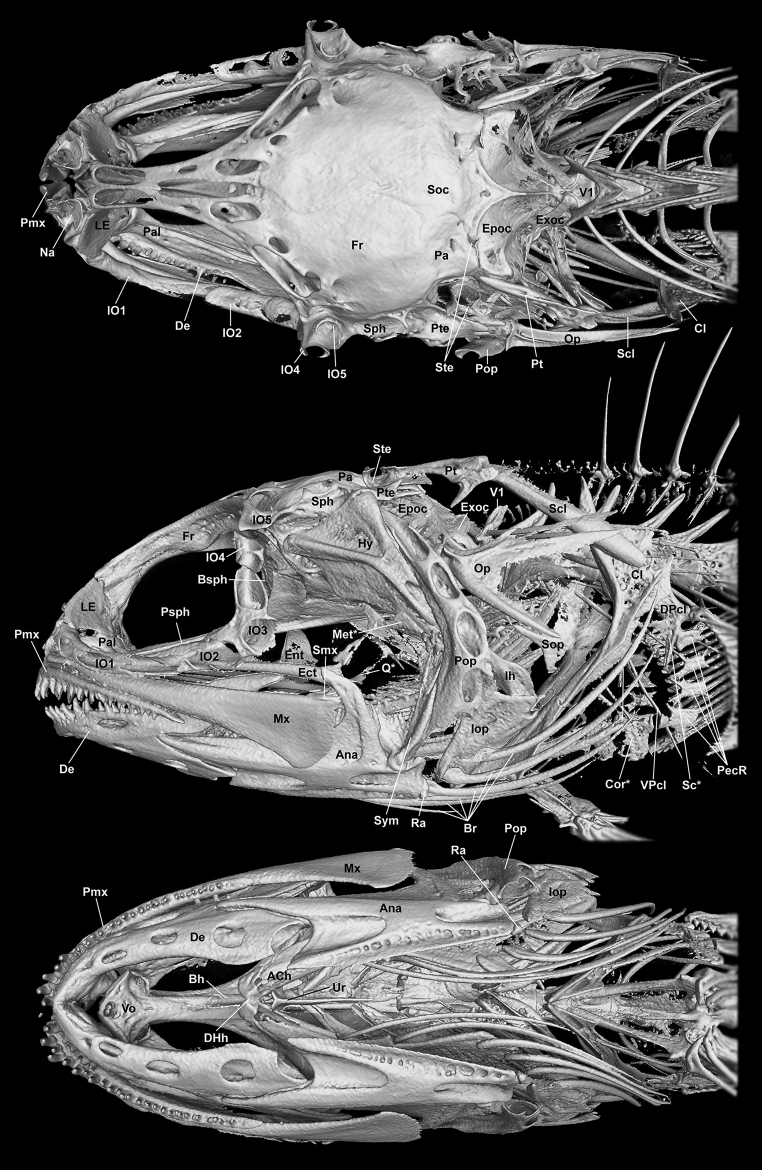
Three-dimensional reconstruction of head and anterior body in *Opistognathusctenion* (KAUM–I. 174226, 30.6 mm SL), based on CT scanning. Photographed by G. Shinohara and K. Fujiwara. Abbreviations: ACh, anterior ceratohyal; Ana, anguloarticular; Bh, basihyal; Br, branchiostegal rays; Bsph, basisphenoid; Cl, cleithrum; Cor, coracoid; De, dentary; DHh, dorsal hypohyal; DPcl, dorsal postcleithrum; Ect, ectopterygoid; Ent, entopterygoid; Epoc, epiotic; Exoc, exoccipital; Fr, frontal; Hy, hyomandibular; Ih, interhyal, IO1 to IO5, 1^st^ to 5^th^ infraorbitals, respectively; Iop, interopercle; LE, lateral ethmoid; Met, metapterygoid; Mx, maxilla; Na, nasal; Op, opercle; Pa, parietal; Pal, palatine; PecR, pectoral radial; Pmx, premaxilla; Pop, preopercle; Psph, parasphenoid; Pt, posttemporal; Pte, pterotic; Q, quadrate; Ra, retroarticular; Sc, scapula; Scl, supracleithrum; Smx, supramaxilla; Soc, supraoccipital; Sop, subopercle; Sph, sphenotic; Ste, supratemporal; Sym, symplectic; Ur, urohyal; V1, 1^st^ vertebral centrum; Vo, vomer; and VPcl, ventral postcleithrum. Asterisks indicate poorly resolved features.
